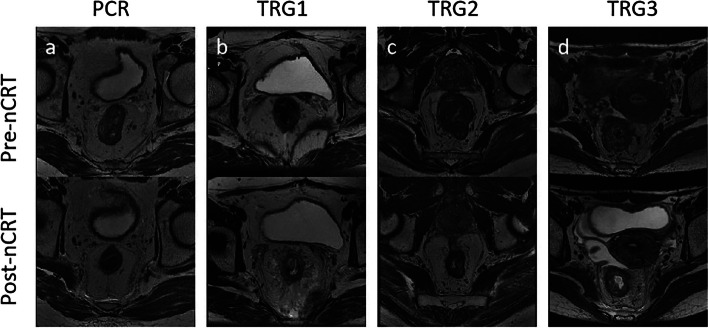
T2-weighted MRI images on pre-nCRT and post-nCRT. a pCR. b TRG1. c TRG2. d TRG3
